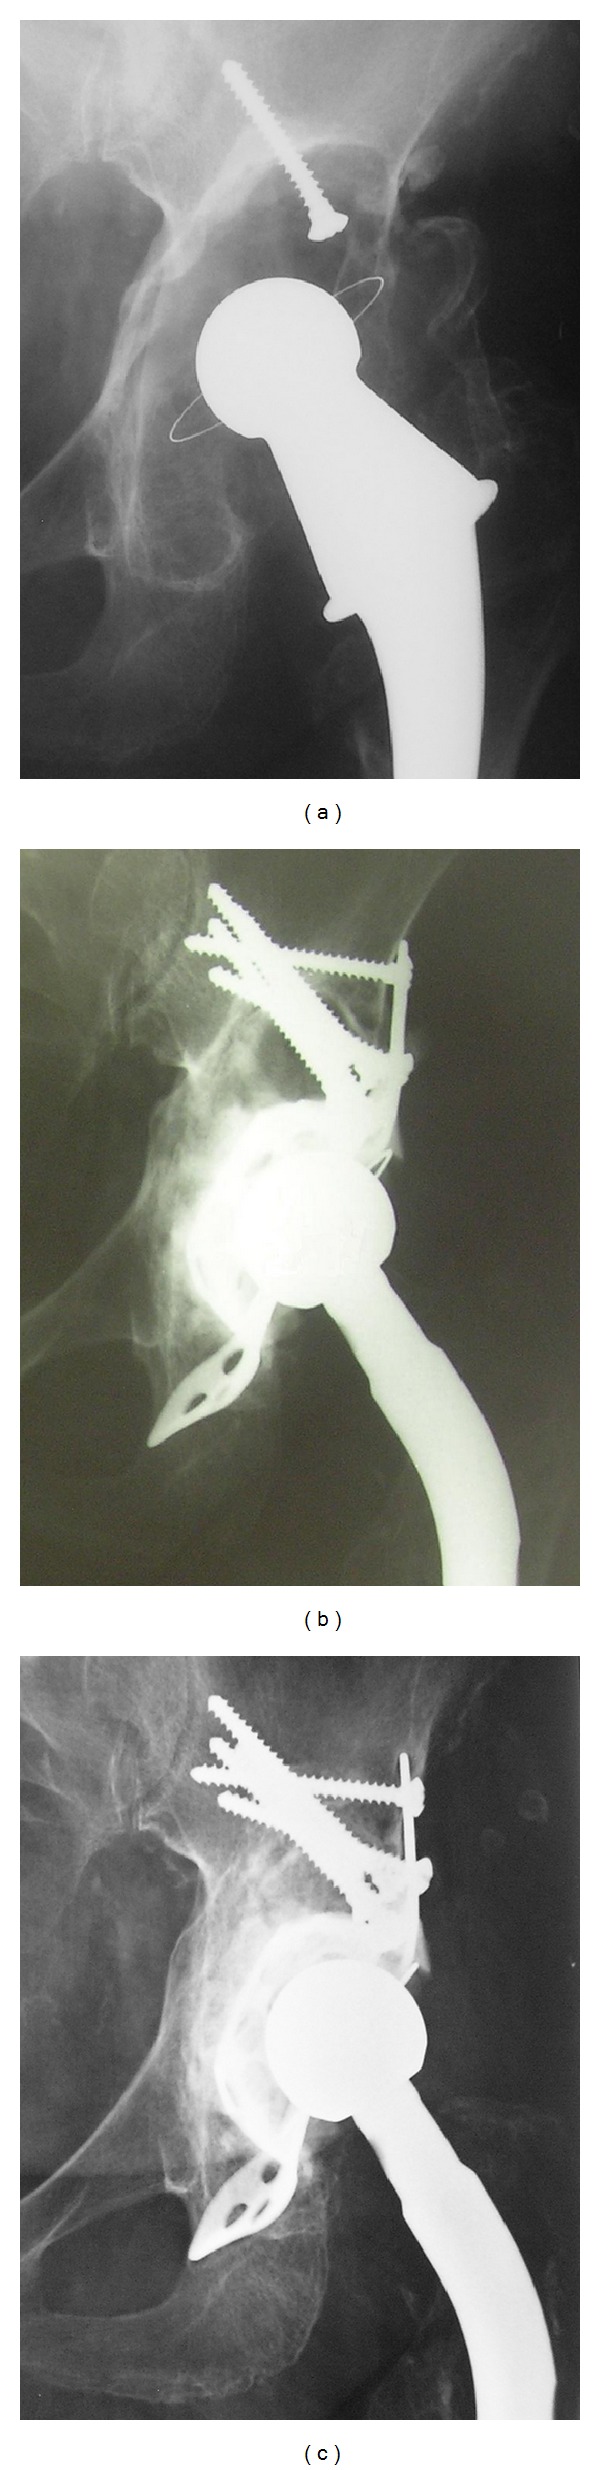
Preoperative X-ray of a 71-year-old female patient with extensive acetabular bone loss (type IIIB) around cemented cup (a). Radiograph after revision with the Burch-Schneider APC and structural allograft (b). Fourteen-year follow-up shows the stability of the reconstruction cage and the incorporation of the bone graft (c).
